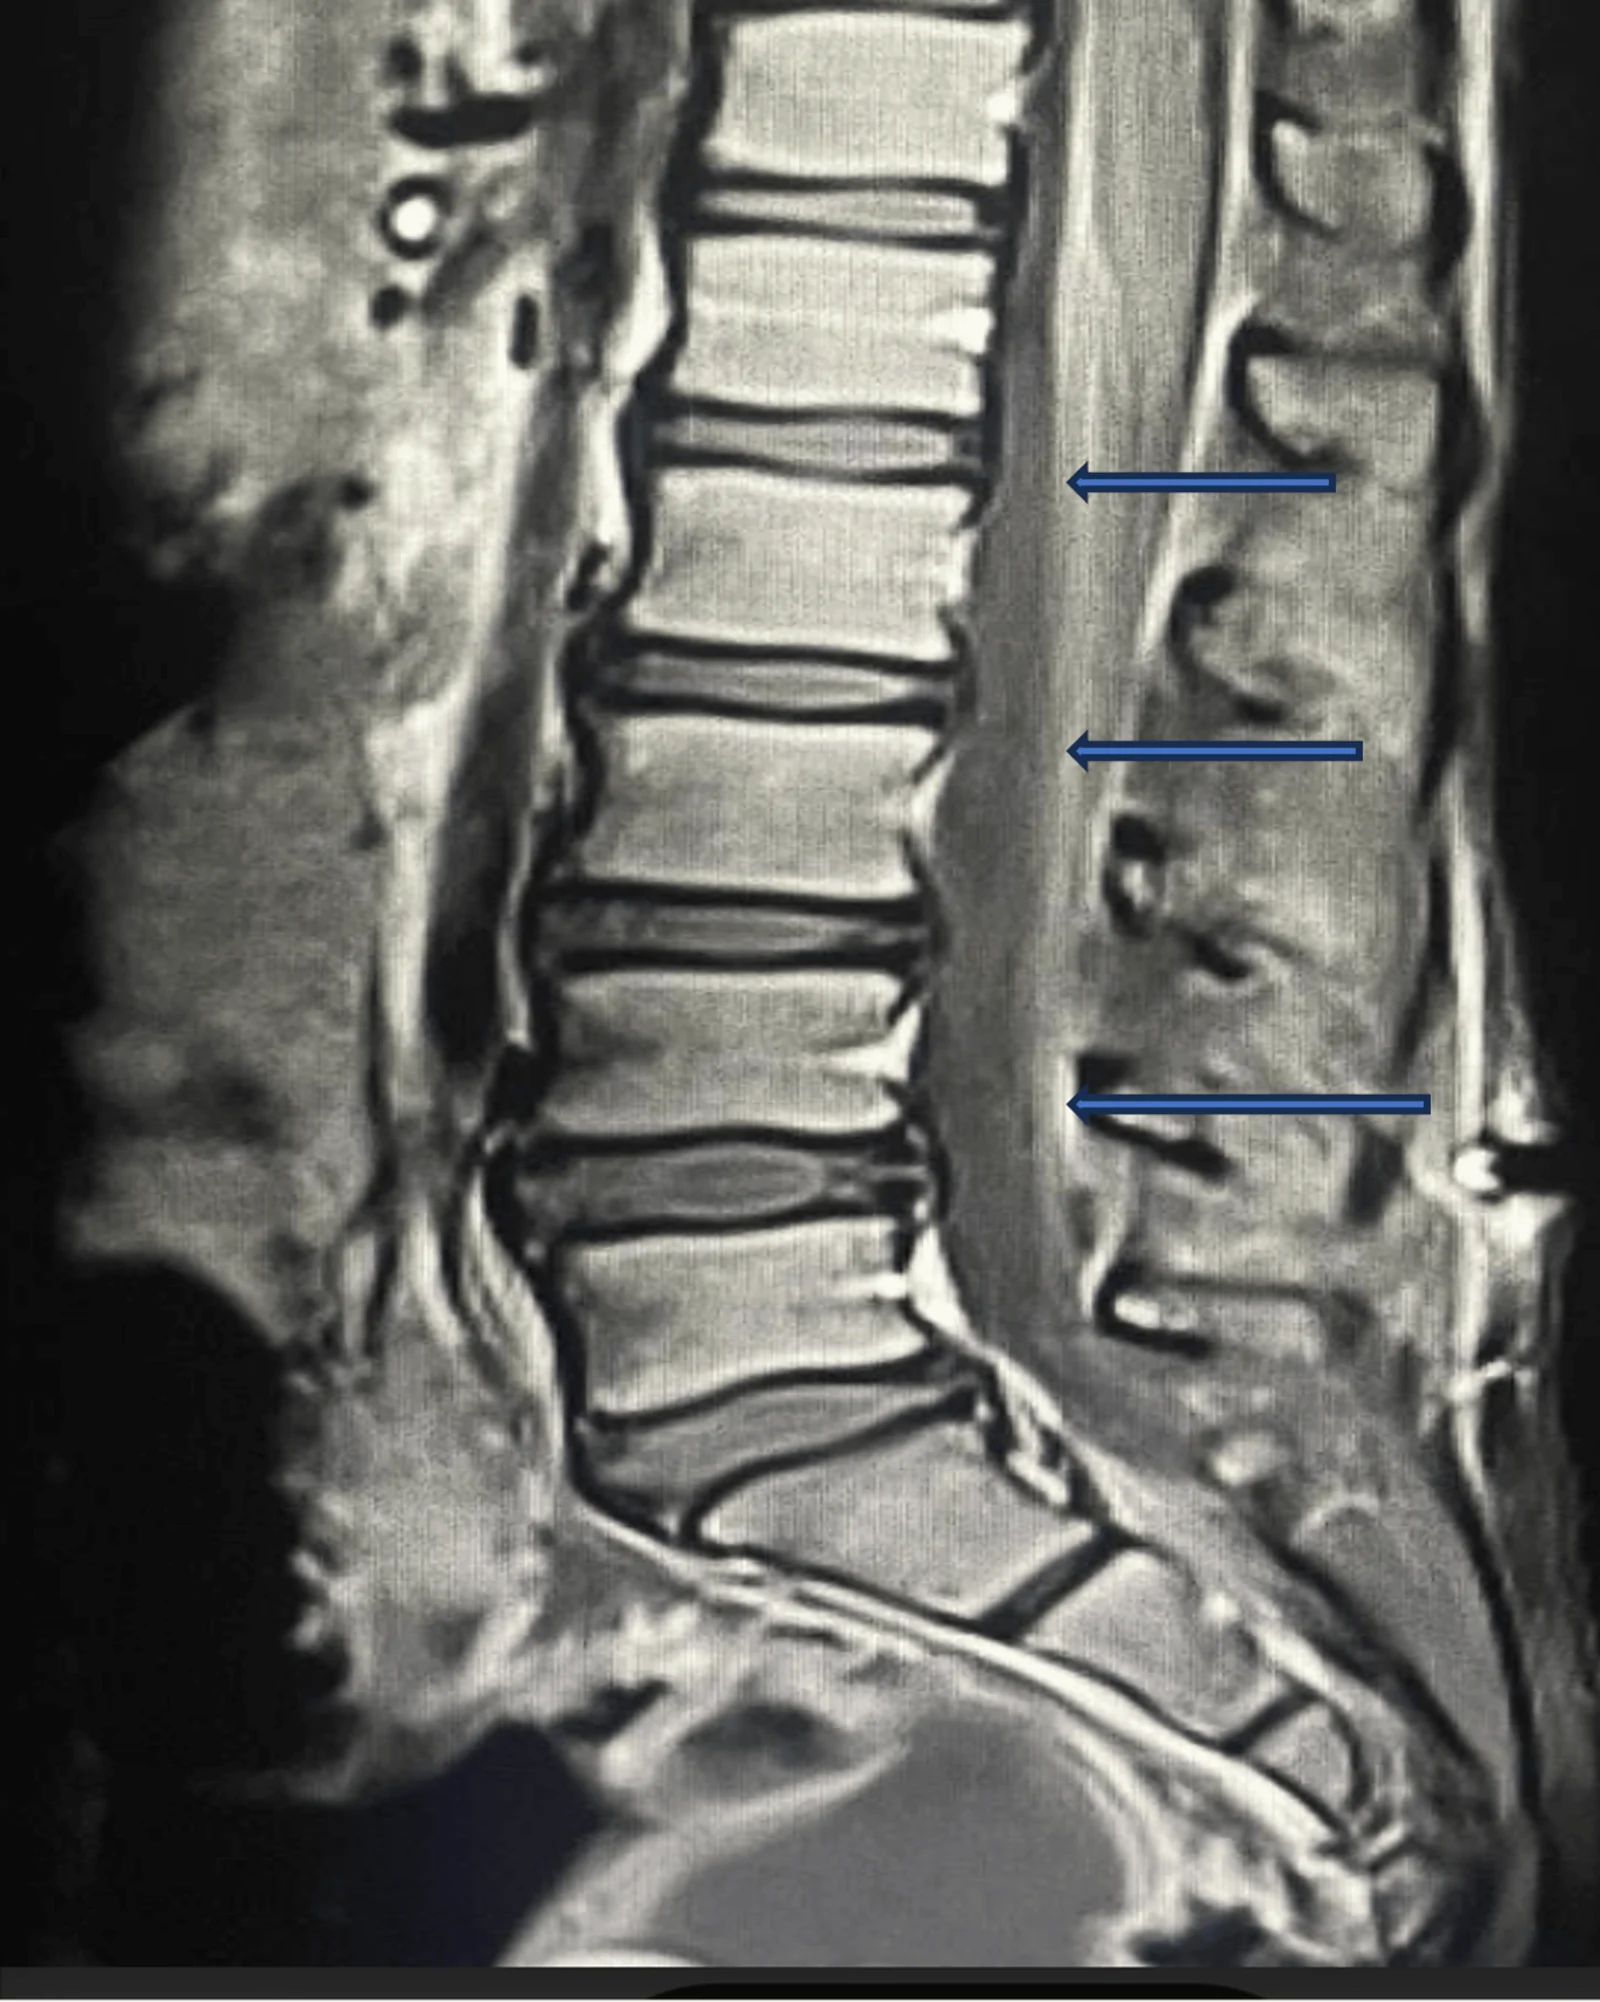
Spinal MRI showing enhancement of the cauda equina nerve roots (blue arrows), a sign of radiculoneuritis
